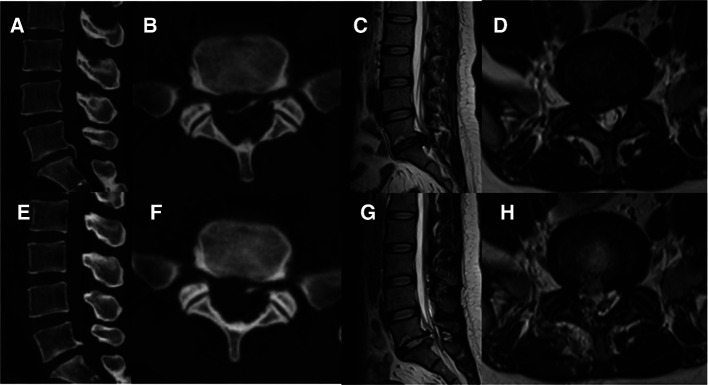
Pre- and post-operative CT and MRI (A-H). (A-D) Preoperative CT and MRI revealed lumbar disc herniation combined with calcification. (E-H) Postoperative CT and MRI revealed that the calcified intervertebral disc was removed and the compressed nerve root had been relieved by PEID.
